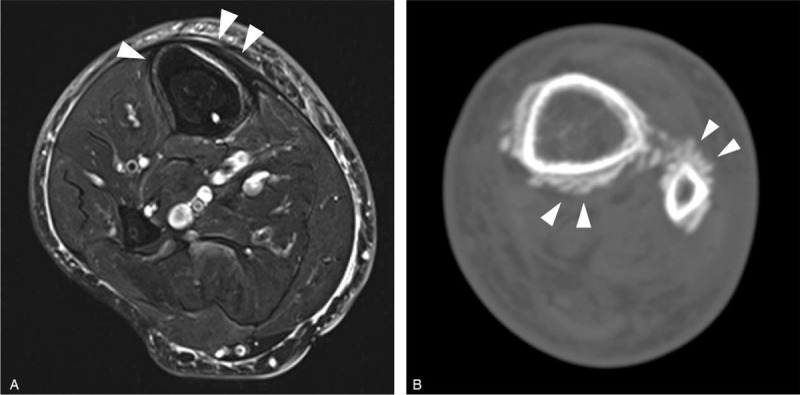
(A) Axial fat-suppressed T2-weighted image of the right lower leg demonstrates a periosteal thickening with mineralization at the anterior aspect of the tibia, suggesting a single lamellar periosteal reaction (arrowheads). (B) CT of the left ankle demonstrates spiculated periosteal reactions at the distal tibia and fibula (arrowheads). No bone or soft tissue mass lesions or cortical destruction were noted.
